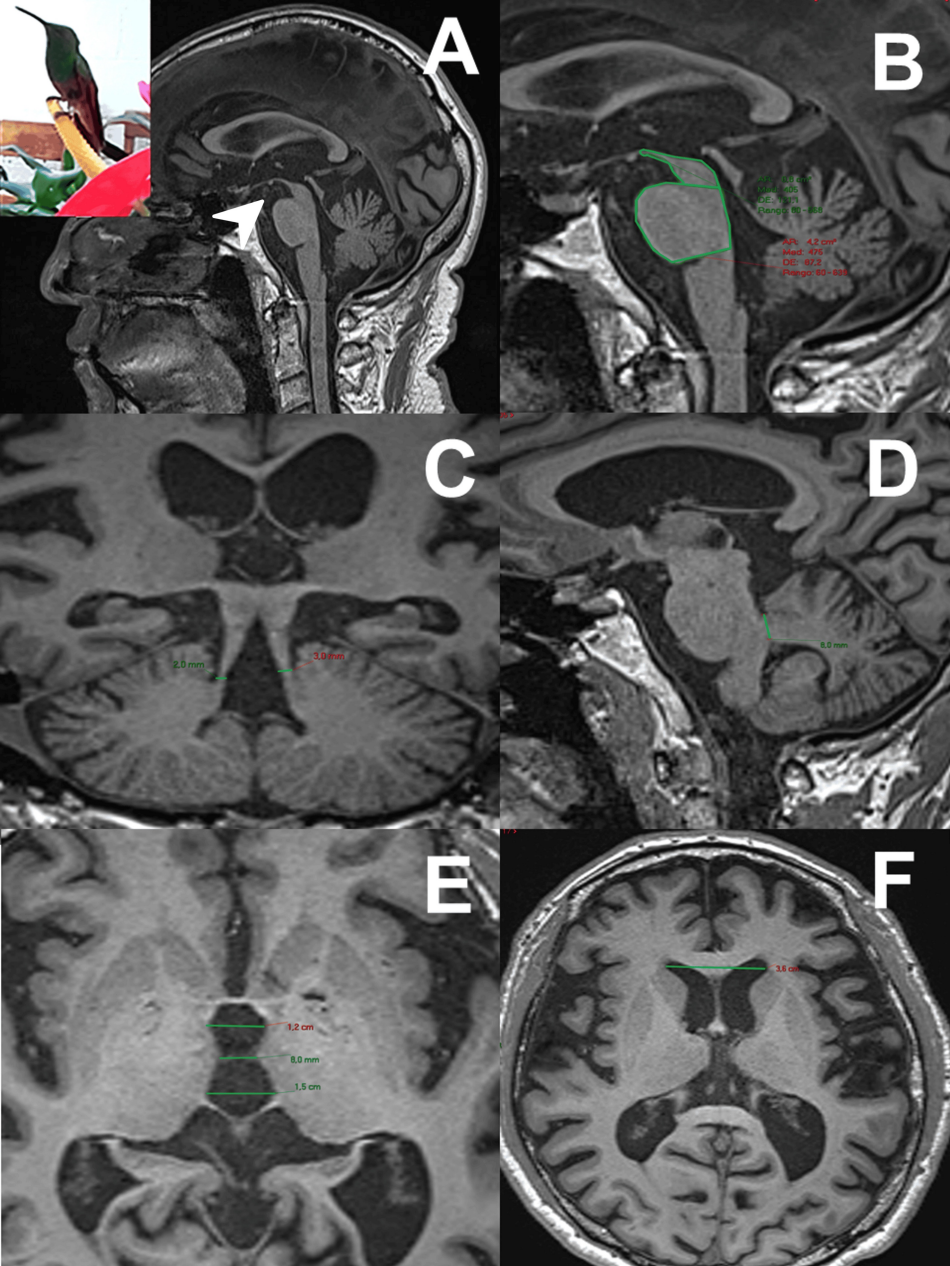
MRI visual assessment and planimetric measurements for MRPI and MRPI 2.0 calculation. A: hummingbird sign (arrow); B: sagittal midbrain area and sagittal pons area; C: coronal superior cerebellar peduncle width; D: sagittal medial cerebellar peduncle width; E: third ventricle; F: frontal horns of lateral ventricles. The hummingbird image used is derived from one of the patient cases included in our series, and the hummingbird photography was taken by Dr. Boll with a personal camera; both are original (not externally sourced). MRPI: magnetic resonance Parkinsonism index
